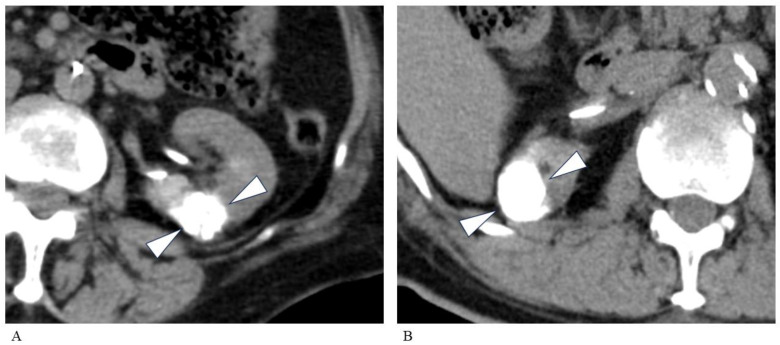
Comparison of different embolic materials. (A) A CT image of a 78-year-old woman with a left RCC. This image was taken immediately after TAE (mixture of absolute ethanol and iodized oil). A 19 mm RCC is clearly seen (arrowheads). The accumulation score was 3 (good). (B) A CT image of a 79-year-old man with a right RCC. A 23 mm RCC is clearly seen (arrowheads) after TAE (injection of iodized oil followed by gelatin sponge). There is no apparent difference in iodized oil accumulation compared with the TAE using absolute ethanol. The accumulation score was 3 (good). CT, computed tomography; RCC, renal cell carcinoma; TAE, transarterial embolization.
